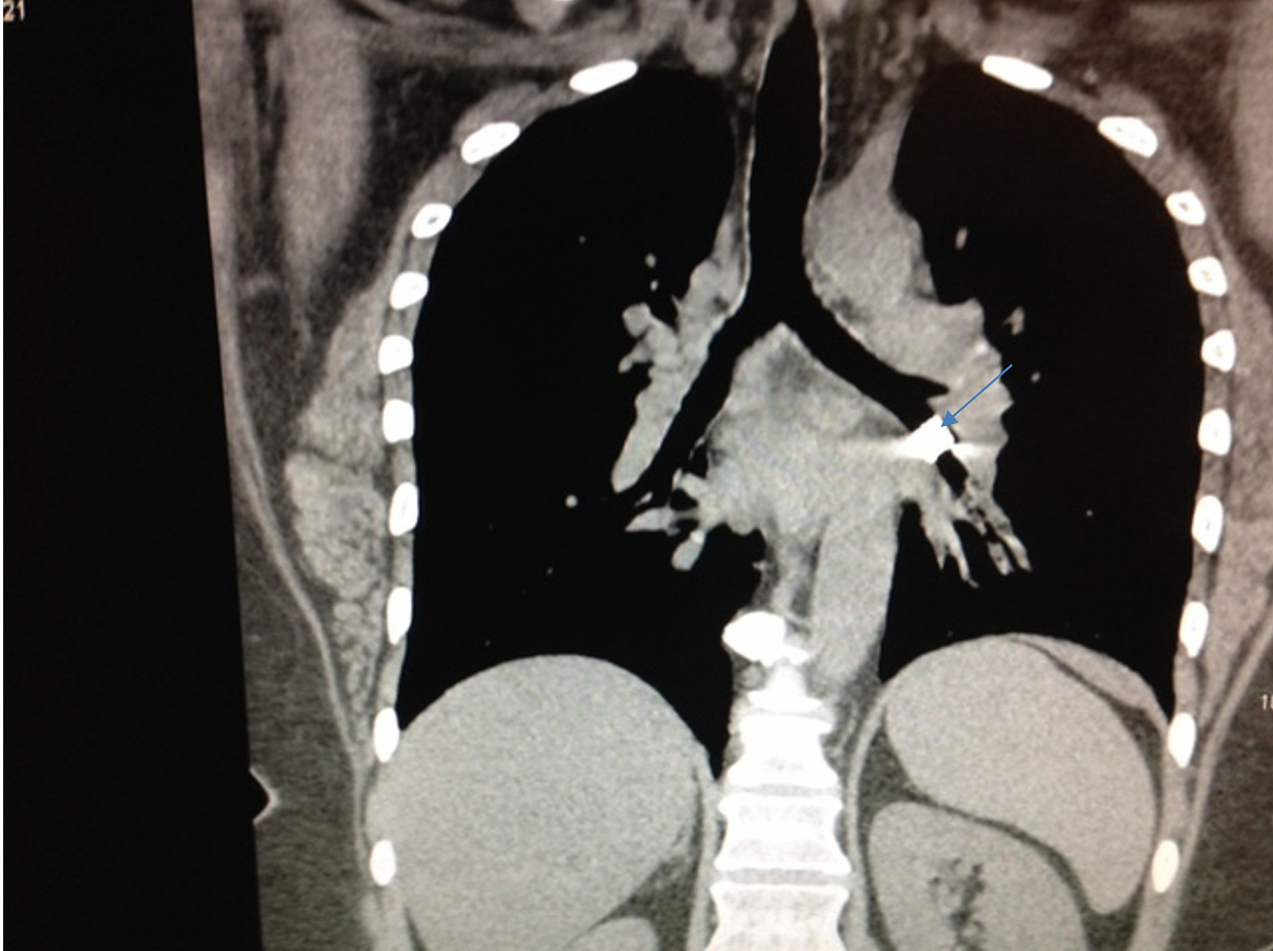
CT scan of the chest showing the metallic pipe in the left lung just after the takeoff of the upper lobe as marked by the arrow. CT, computed tomography
